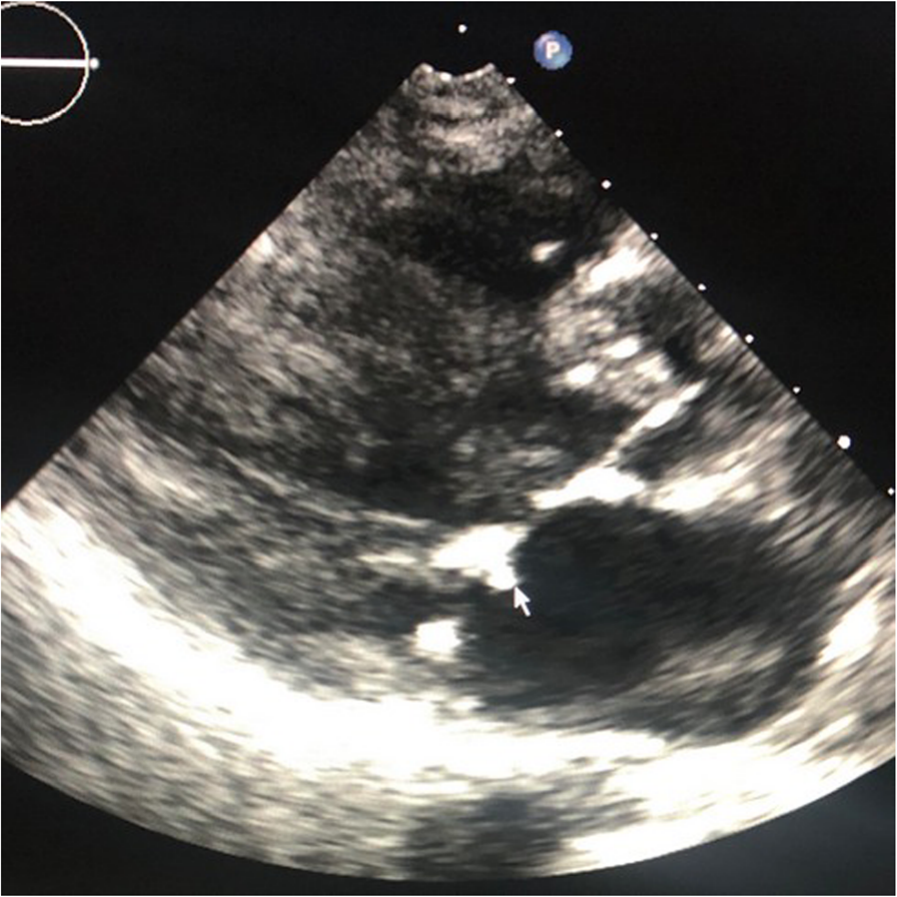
2-D Transthoracic echocardiogram. Parasternal long axis showing vegetation on mitral valve. Indicated by arrow
